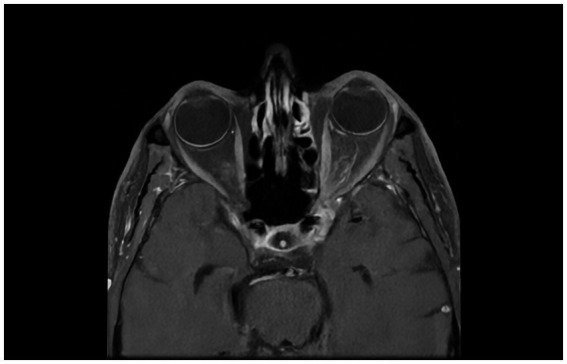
Axial T1-weighted, fat-suppressed, post-contrast MRI of the brain and orbits show enhancement in the left orbital apex involving the optic nerve sheath, without well-defined nodularity. The enhancement extends to the superior orbital fissure, the anterior aspect of the cavernous sinus, the pterygopalatine fossa, and the inferior orbital fissure.
